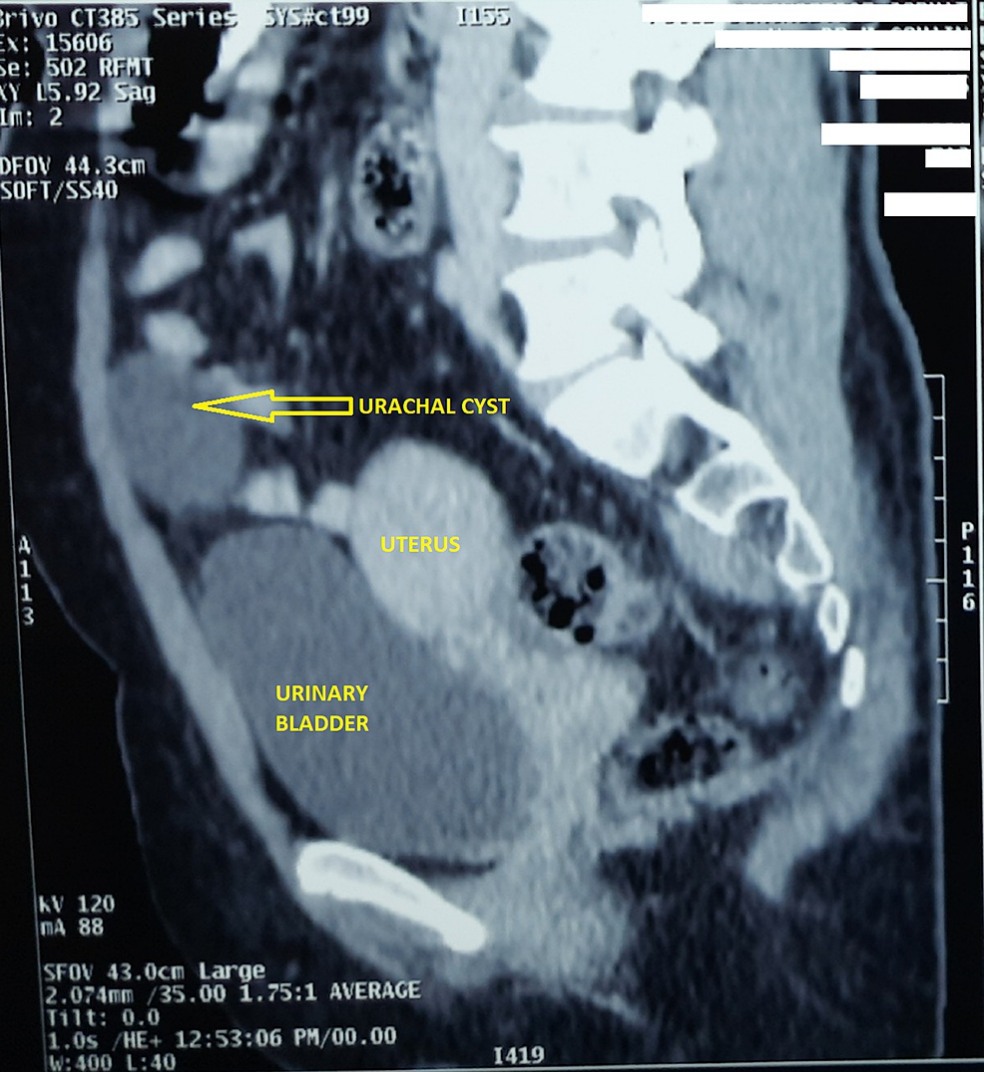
CT scan abdomen showing urachal cyst Abbreviation: CT, computed tomography.
